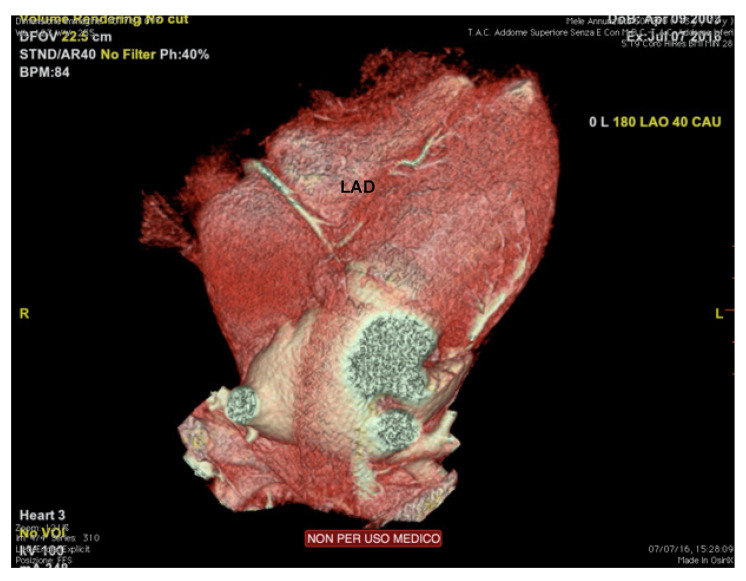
Cardiac computed tomography of an endurance athlete (runner) symptomatic for syncope. The figure shows an intramural course of the left anterior descending coronary artery. LAD: left anterior descending.
